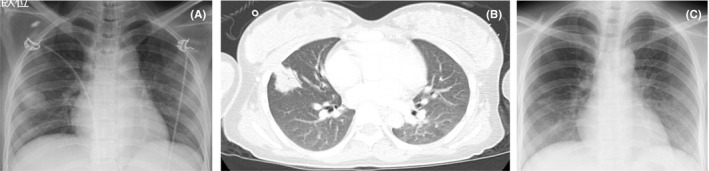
A, Chest radiograph, showing a 2.1 × 2.6 cm^2^ nodule in the right lung (white arrowheads). B, Chest computed tomography, showing a 2.6 × 2.7 cm^2^ irregular nodule with air bronchogram in the right S4 (white arrowheads). C, Chest radiograph, showing the diminution of the lung nodule 30 d after treatment initiation
